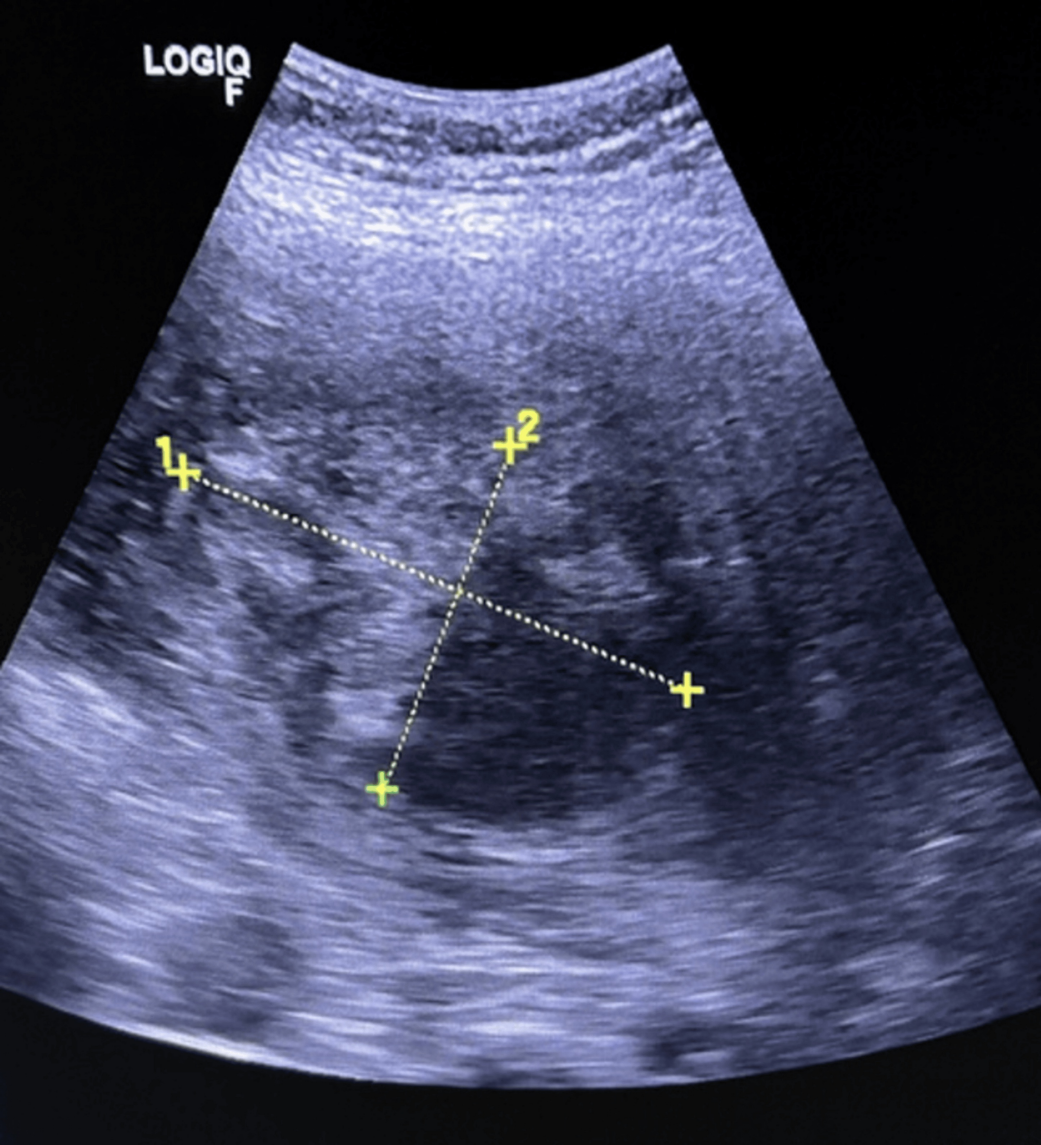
Grey-scale ultrasound image showing a well-defined iso-echogenic lesion in the uterus, measuring 11.5 x 8.8 x 6.3 cm, with a cystic area within, suggestive of a fibroid with cystic degeneration
